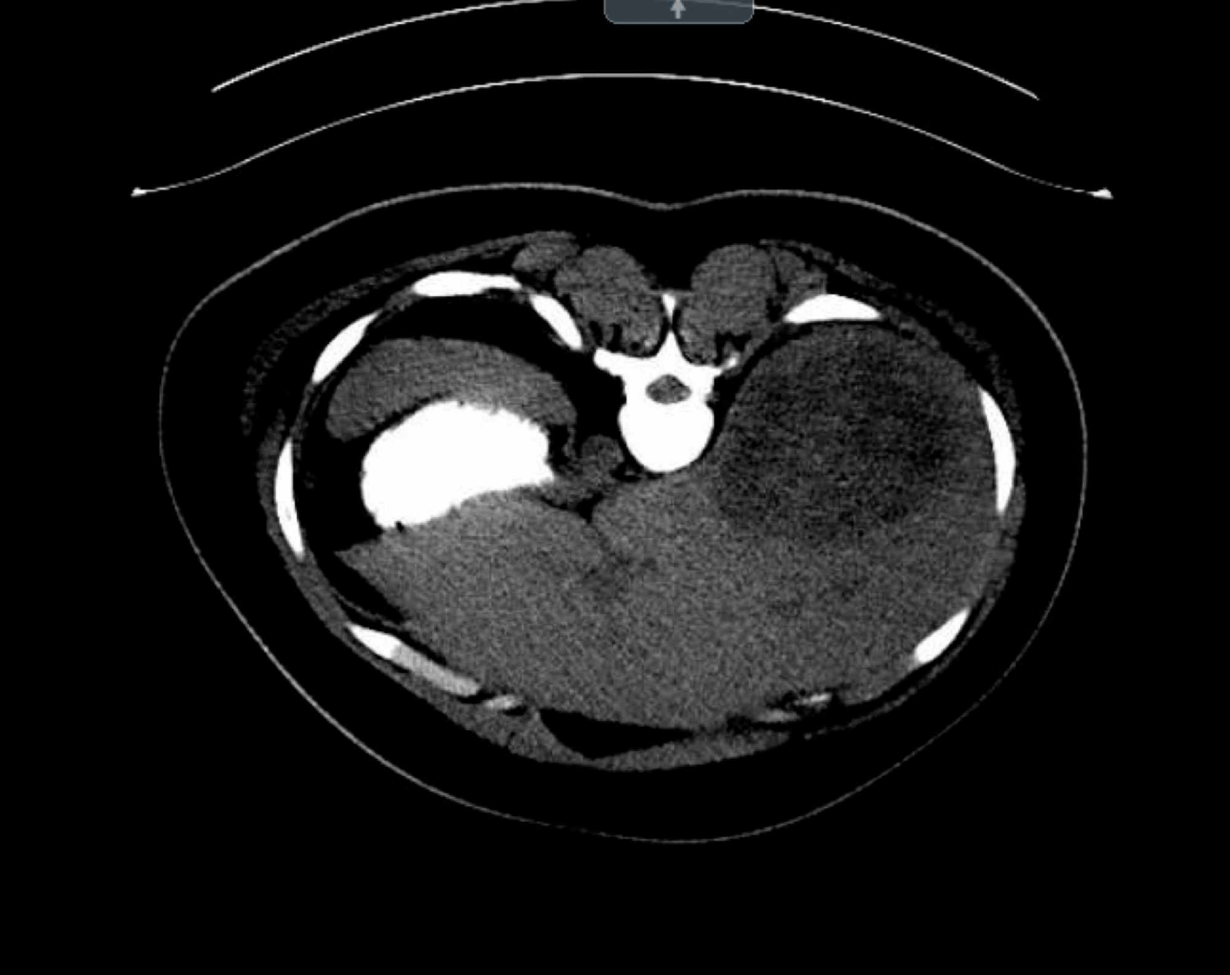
Computed tomography scan of the liver An abdominal computed tomography scan revealed a large right hepatic hypodensity measuring 10 cm x 8.5 cm. There was no intra- or extra-hepatic biliary ductal dilation. The gallbladder was unremarkable.
